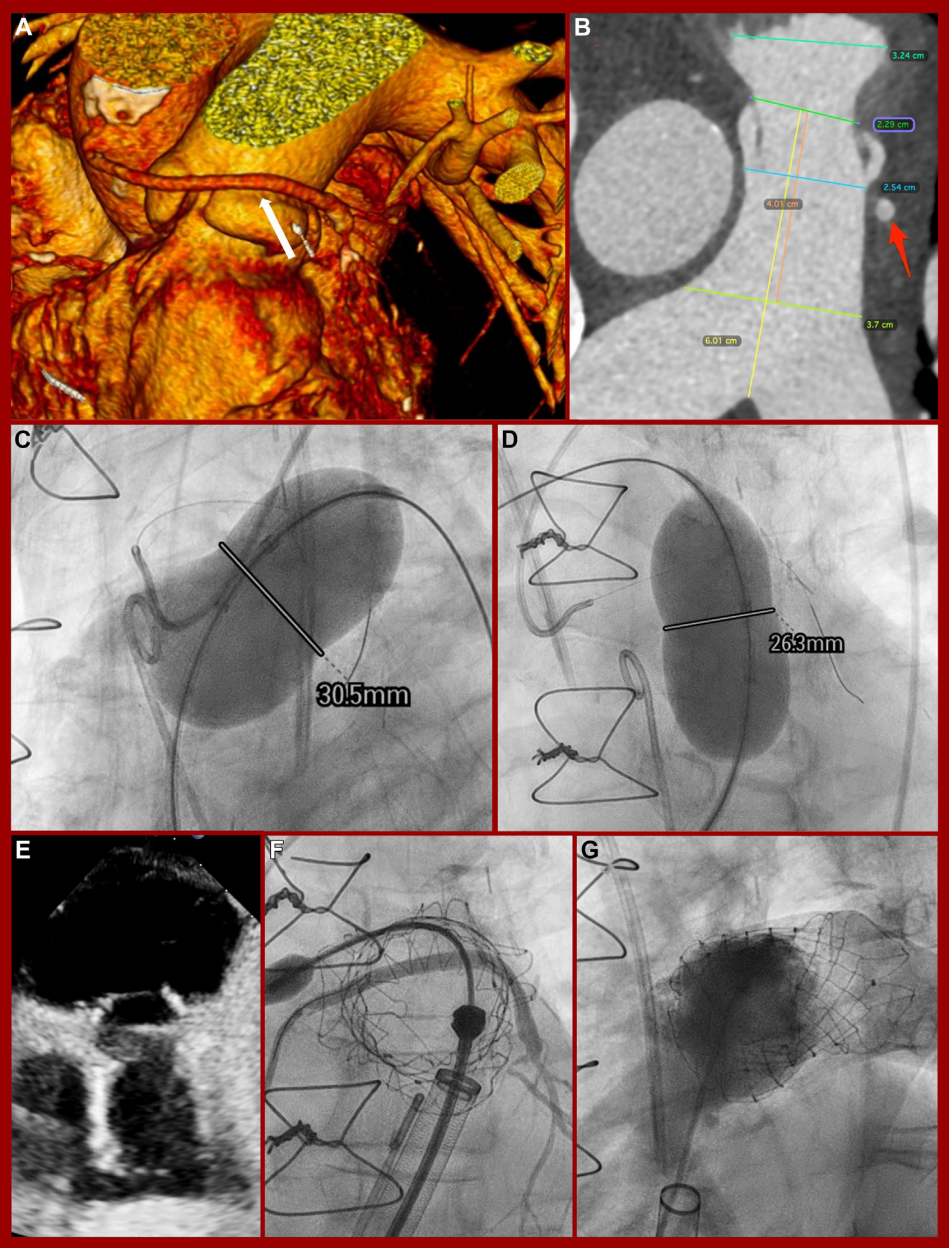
Sizing, Implantation, and Procedural Results of Venus P Valve (A) Coronary venous bypass graft (white arrow). (B) Computed tomography sizing of the main pulmonary artery (red arrow = coronary venous bypass graft). (C and D) Balloon sizing of the main pulmonary artery. (E) Implanted Venus P-Valve. (F) Down-the-barrel view with venous bypass graft contrast. (G) No pulmonary regurgitation in contrast fluoroscopy.
